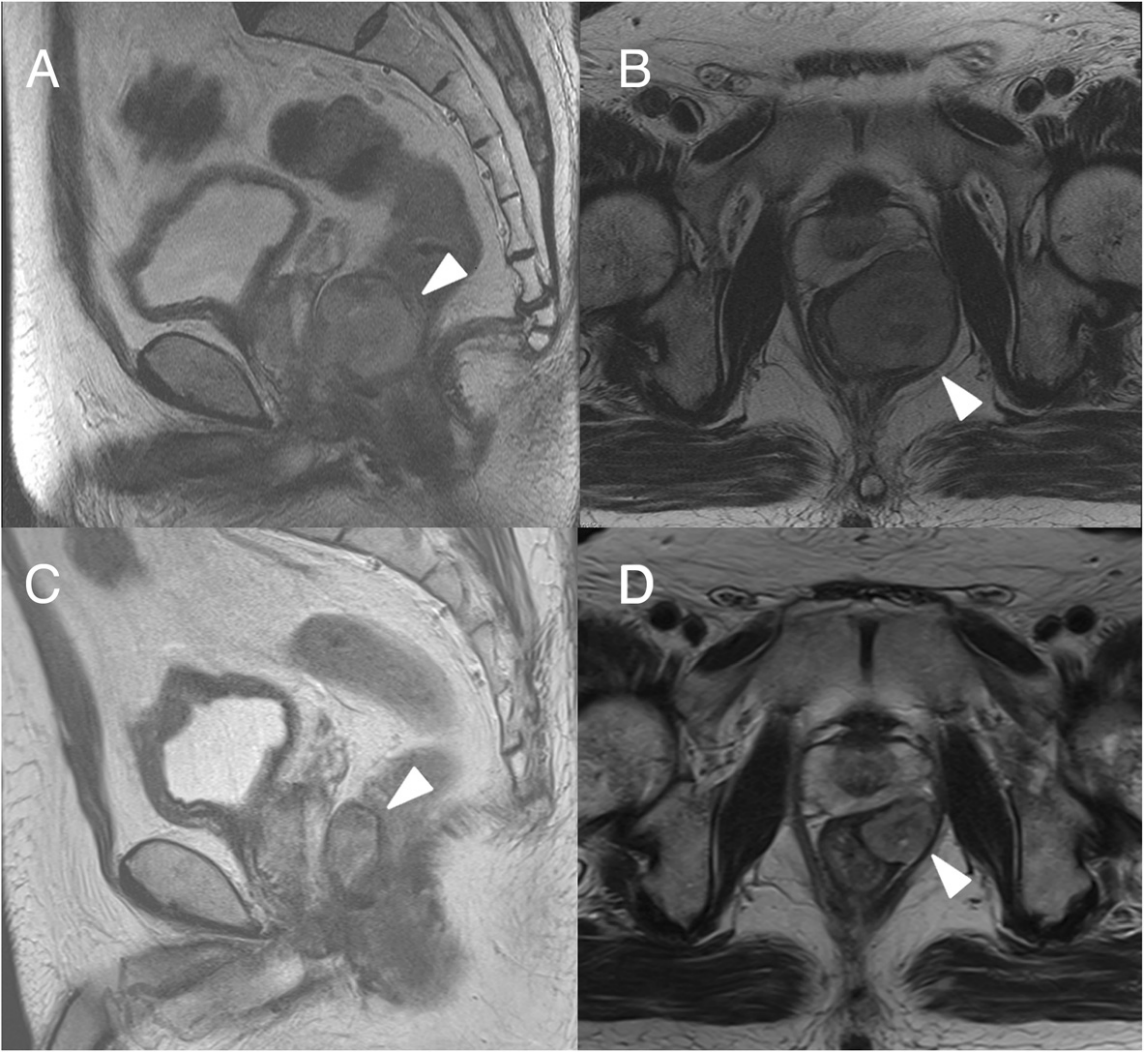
MRI examination. (A, B) Pelvic MRI before imatinib therapy A 45 mm tumor was detected in the lower rectum adjacent to the posterior wall of the prostate (arrowhead). (C, D) Pelvic MRI after imatinib therapy for one year. The tumor shrank to 24 mm in diameter (arrowhead). (A, C: Coronary view, B, D: Sagittal view).
